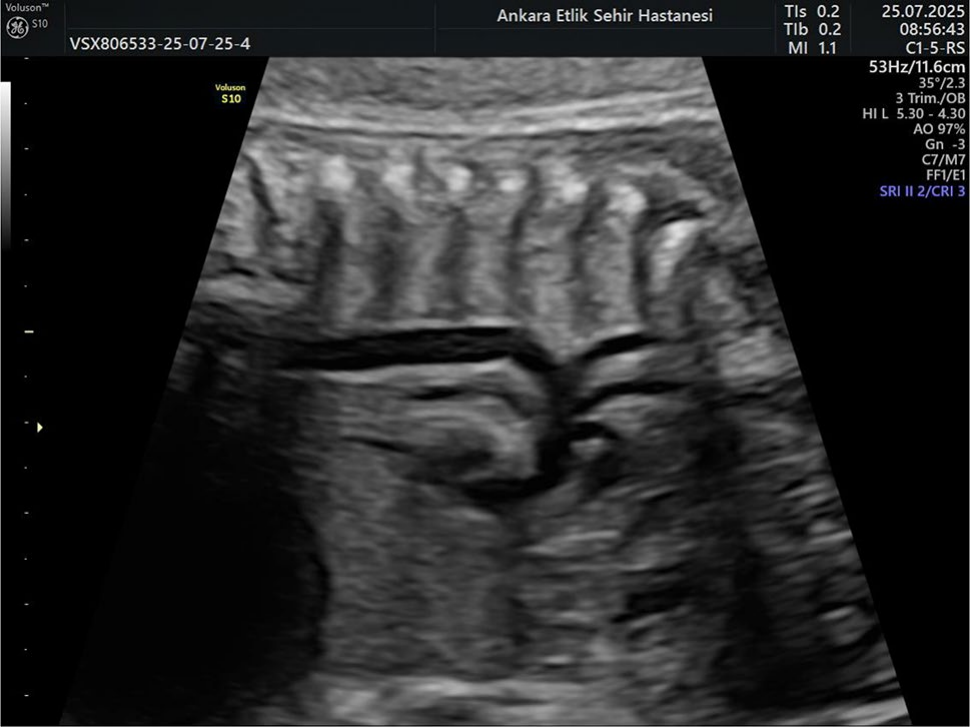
Aortic isthmus in the sagittal plane obtained during third trimester fetal ultrasound
